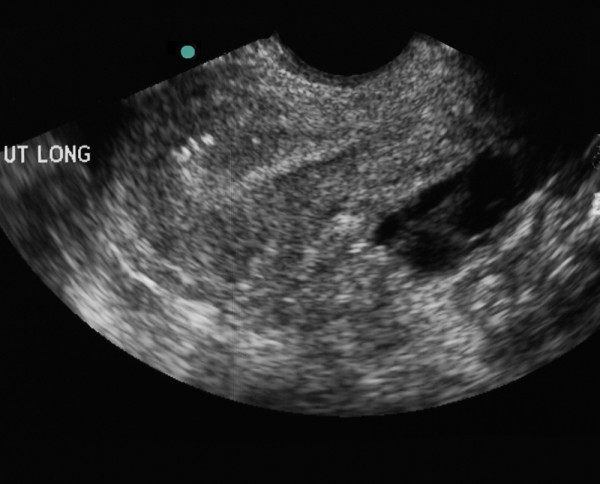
Sagittal view of the uterus on trans-vaginal ultrasound. A bulky heterogeneous anteverted uterus is seen. The striated pattern suggests inflammation and infection.
